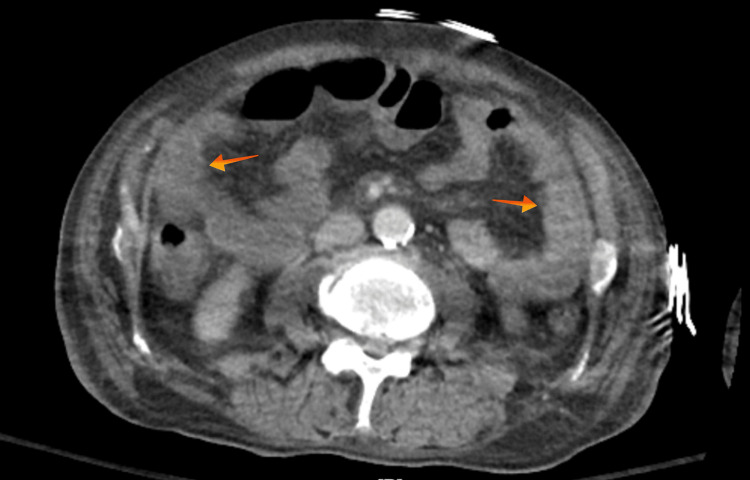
CT of the abdomen and pelvis with IV contrast revealed features of diffuse colitis involving the ascending, descending, and sigmoid colon (arrows).
